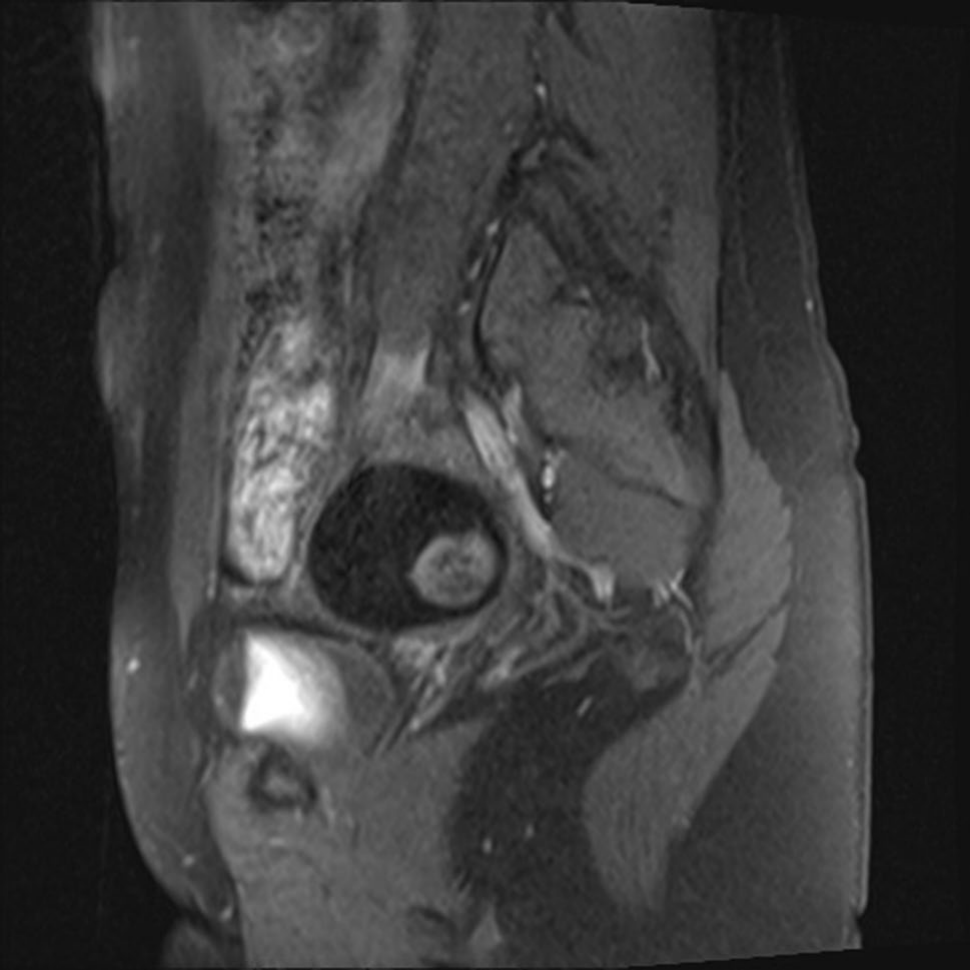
Single ball within a teratoma cyst visible in MRI scan of a 14-year-old patient
